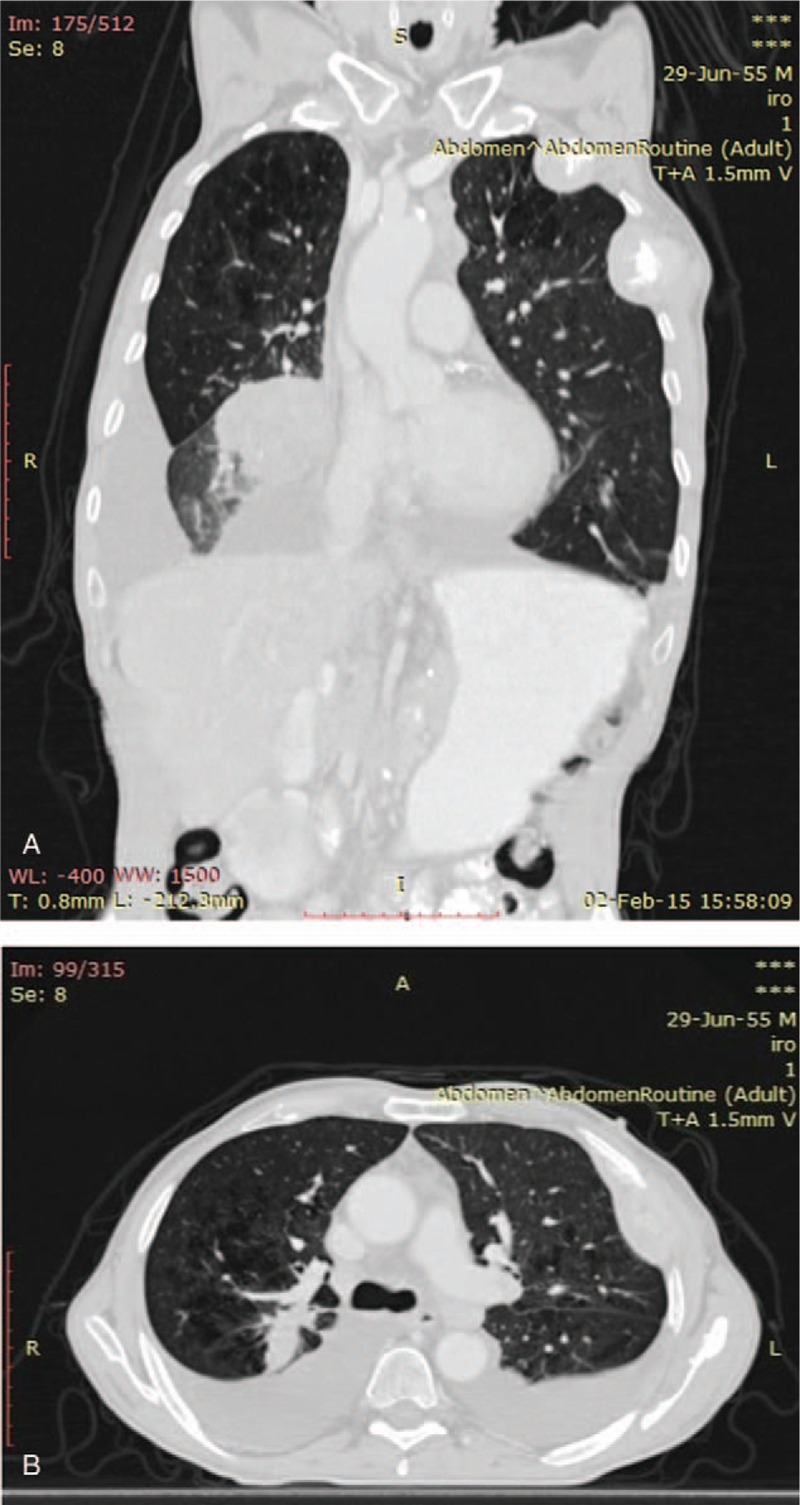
CT scan of the lungs showing progressive disease.
